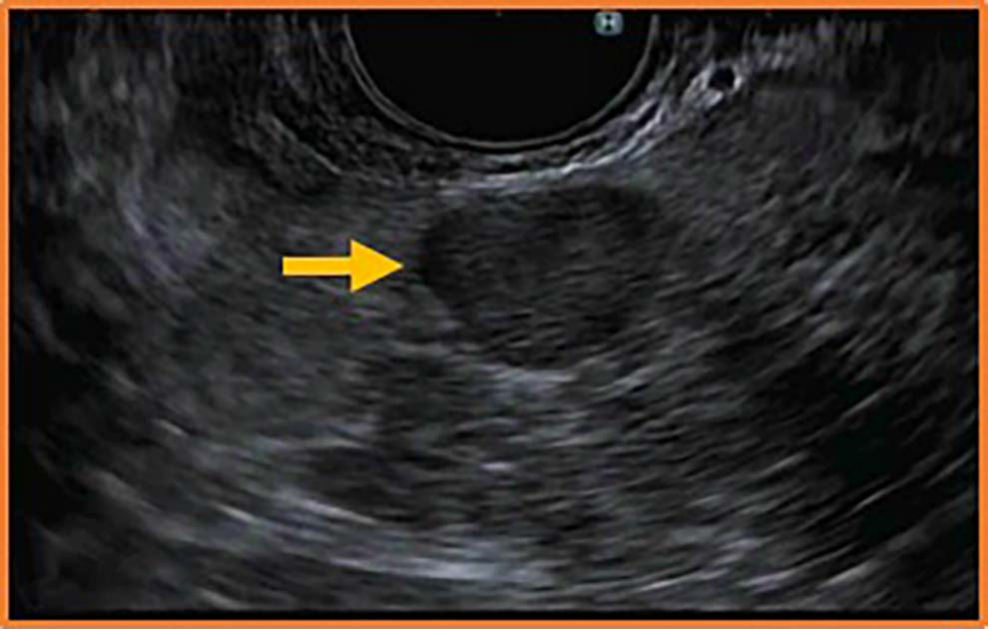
Well-circumscribed hyperechoic lesion of the pancreatic body (arrow) visualized on endoscopic ultrasound.
